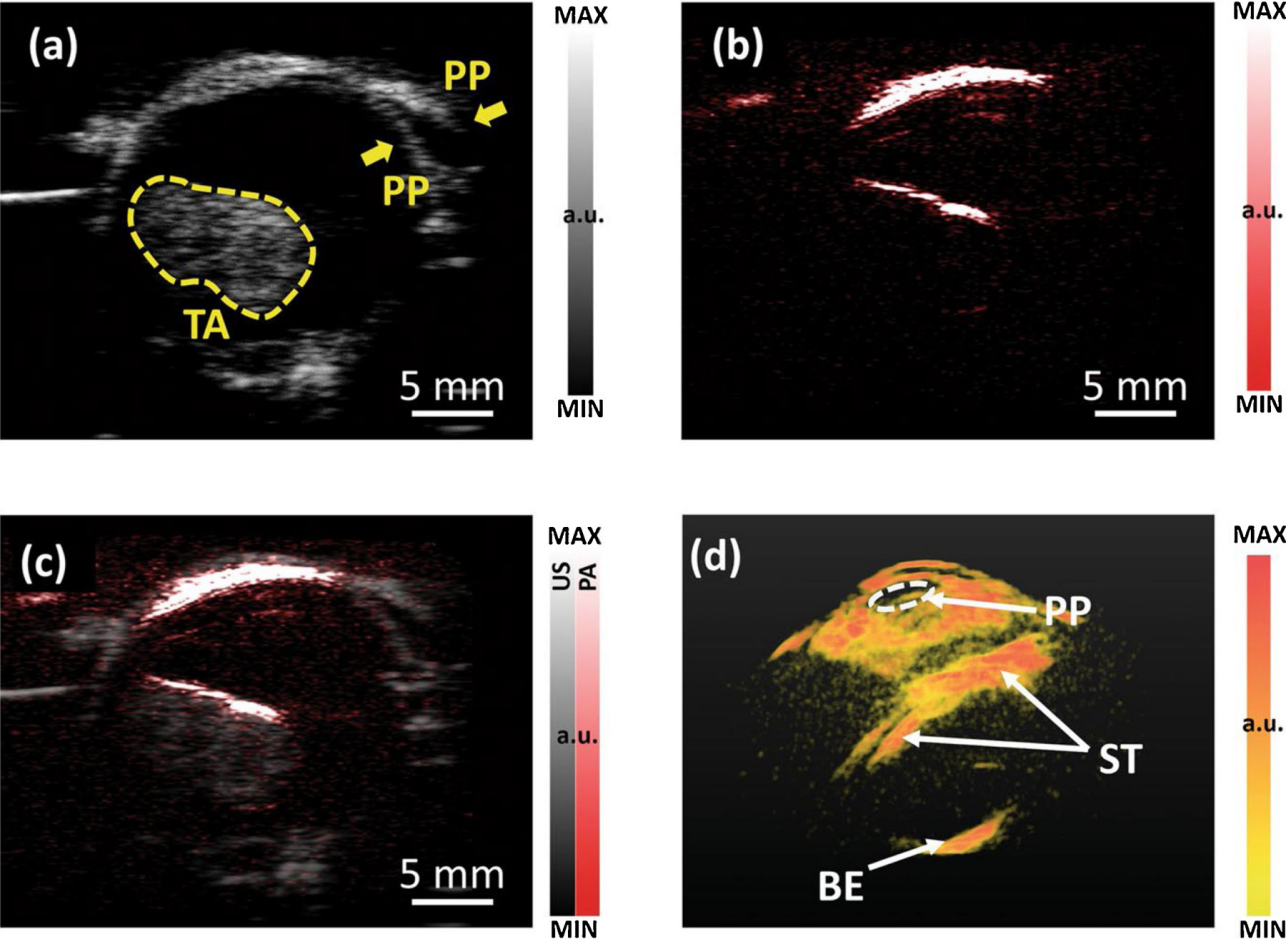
Ultrasound (a), photoacoustic (b), and co-registered (c) B-scan of an ex vivo human ocular globe with a choroidal melanoma tumor. (d) Perspective view of a 3D photoacoustic image of the sample. Parts identified on the images are pupil (PP), tumor area (TA), surface of the tumor (ST), and back of the eye (BE). Reprinted from Ref. [64].
